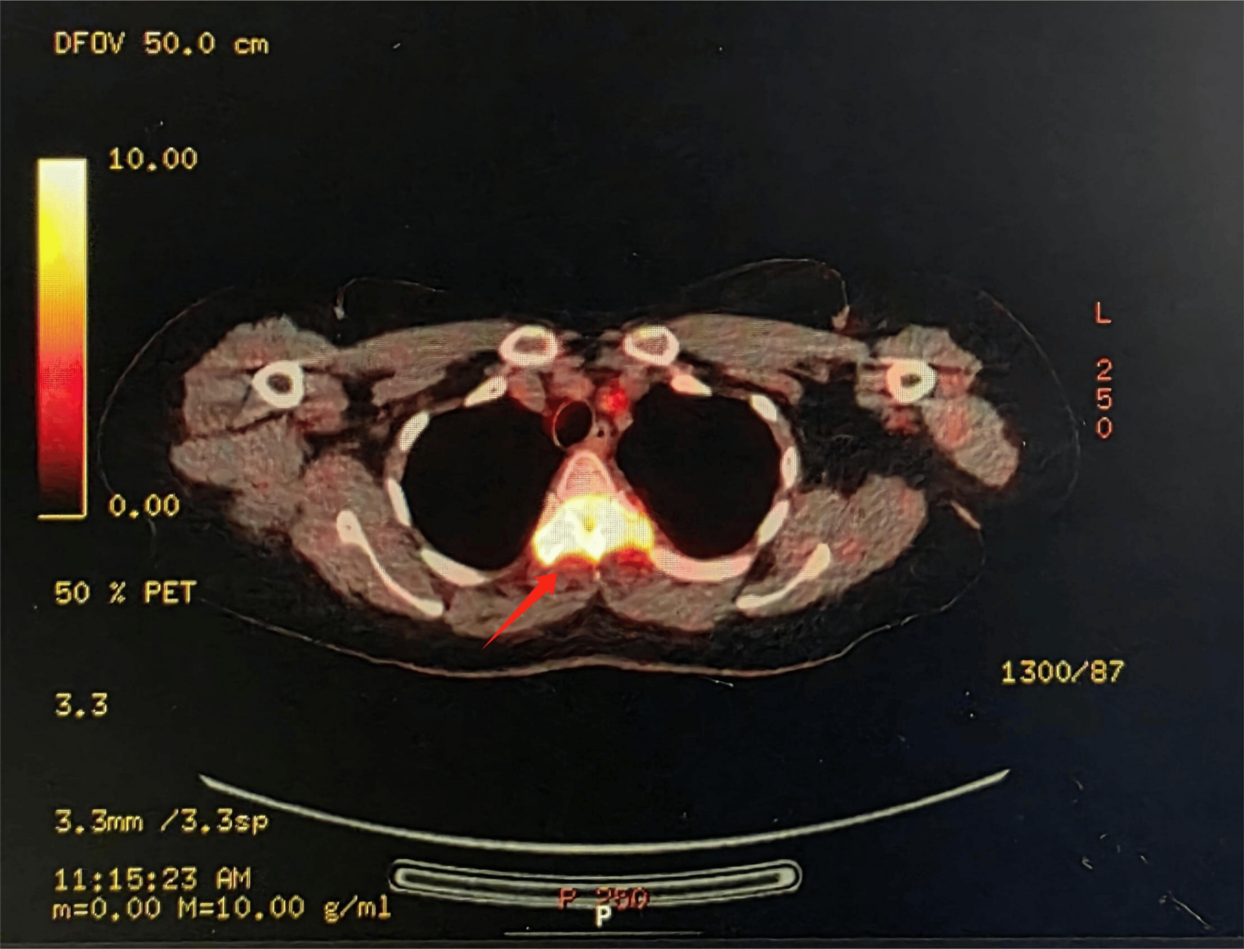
Positron emission tomography (PET)-CT showing hypermetabolic uptake at T4 vertebra (arrow), consistent with active inflammatory or neoplastic process.
